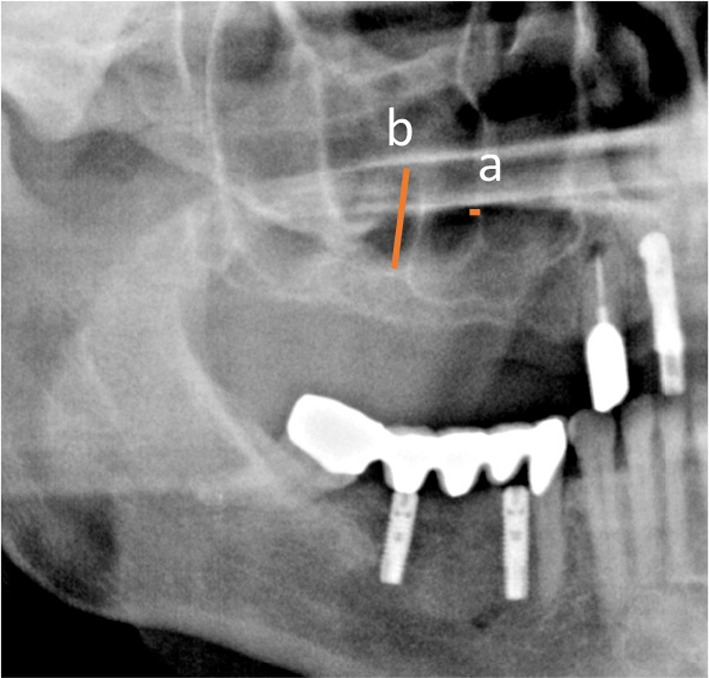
Panoramic radiographic (PR) image of the right maxillary sinus with measurements of the width (a) and height (b) of the septa. On the PR image, only two septa are clearly visible
